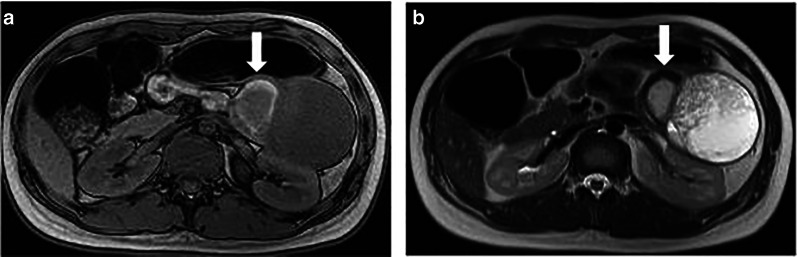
Magnetic resonance imaging. The tumor had bleeding (arrow) shown by high intensity on T1-weighted images (a), and a low intensity on T2-weighted images (b)
